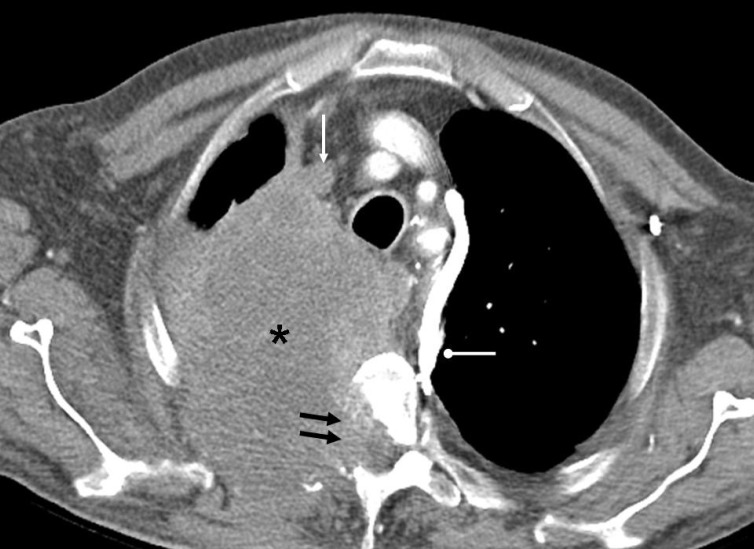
Contrast enhanced CT of the chest of a 64 years old male smoker with a large mass (asterisk) in the apical portion of the right lung shows absence of contrast in the right brachiocephalic vein (white arrow) suggesting tumor thrombus. Other vessels are well opacified. Mass destroys right side of the vertebral body and adjacent rib and extends into the spinal canal (double black arrows). Dilated hemi-azygous vein (oval arrow) is noted along the left side of the mediastinum suggesting collateral flow
